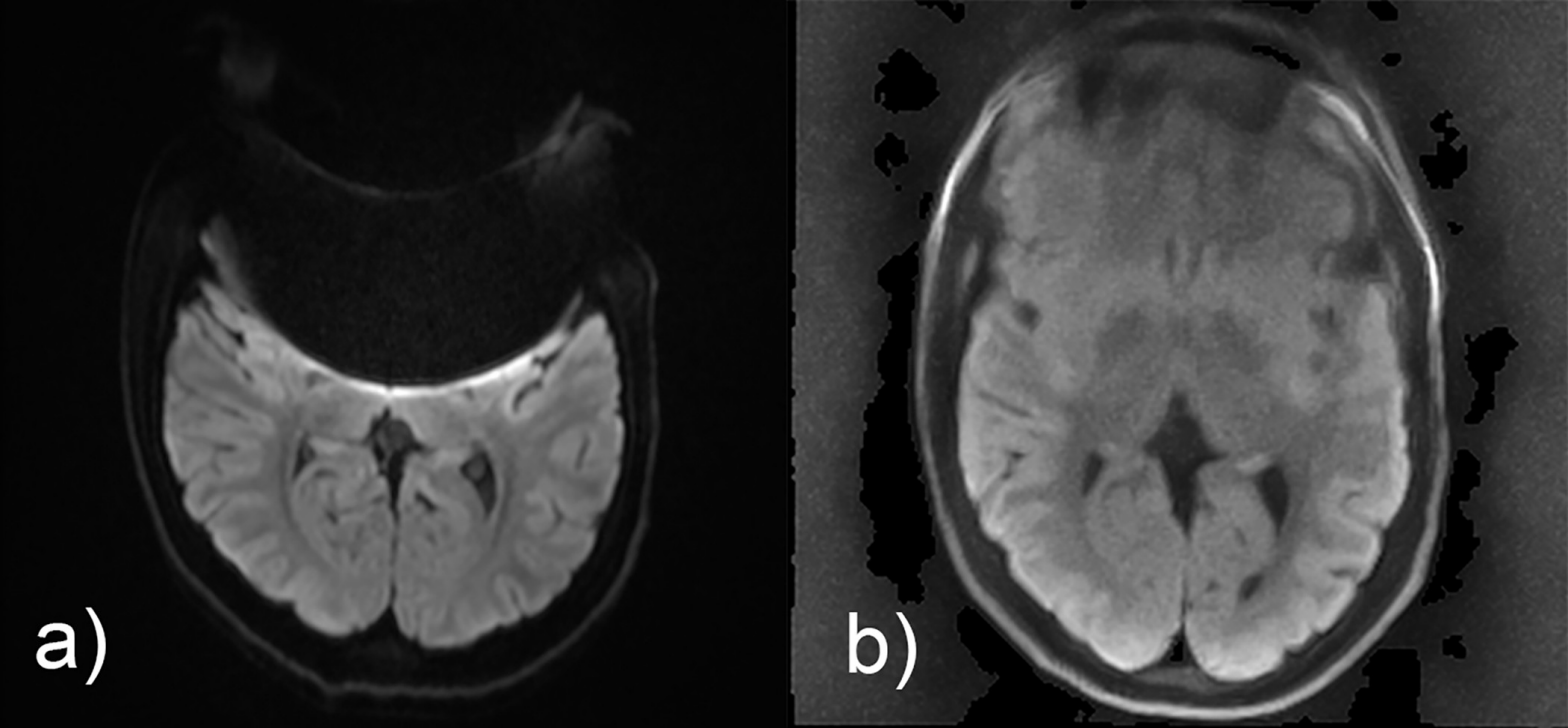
Comparison of isoDW images obtained by (a) EPI-DWI and (b) STEAM-DWI in a 15-year-old adolescent with epilepsy without pathological findings. A brace leads to severe frontal susceptibility artifacts, which are much less pronounced in STEAM-DWI.
